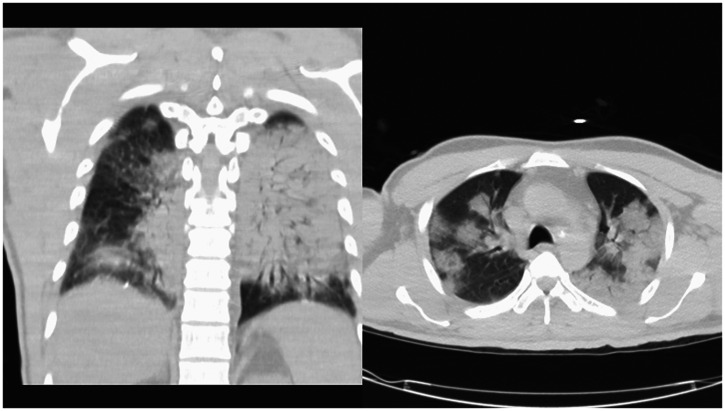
Two views of a computed tomographic scan of the chest without contrast obtained on admission showed extensive bilateral multilobar pneumonia. The diffuse macronodular consolidations and air bronchograms throughout are consistent with bacterial pneumonia.
